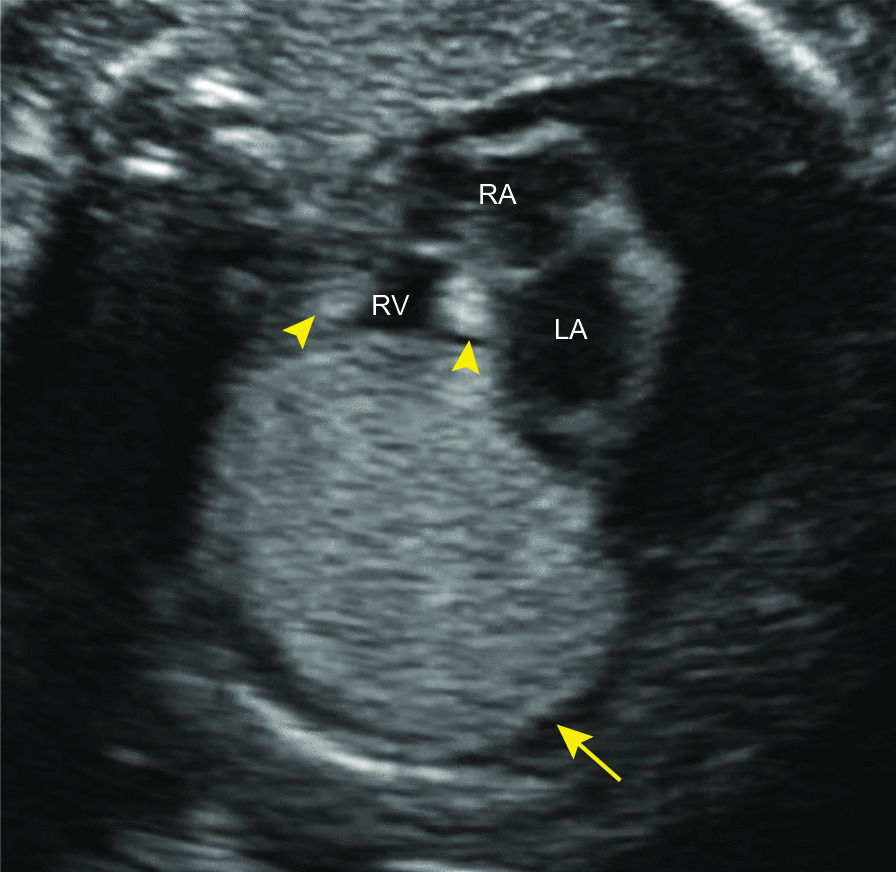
Fetal echocardiography at 26 weeks. A large left ventricular apical mass (arrow) and small nodules in the interventricular septum and right ventricular wall (arrowheads) were observed. *RA* right atrium, *RV* right ventricle, *LA* left atrium
